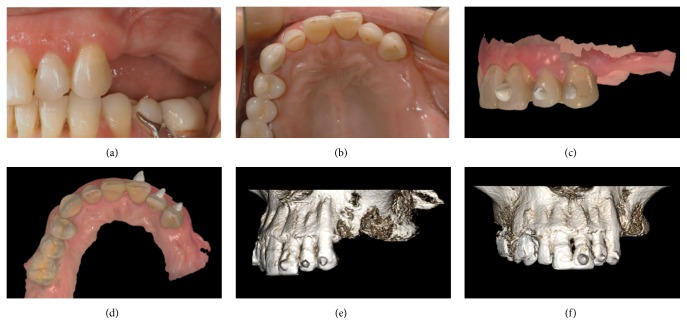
Image acquisition: (a) preoperative clinical picture, side view; (b) preoperative clinical picture, occlusal view; (c) intraoral scan with references, side view; (d) intraoral scan with references, occlusal view; (e) CBCT volume rendering with references, side view; (f) CBCT volume rendering with references, frontal view.
